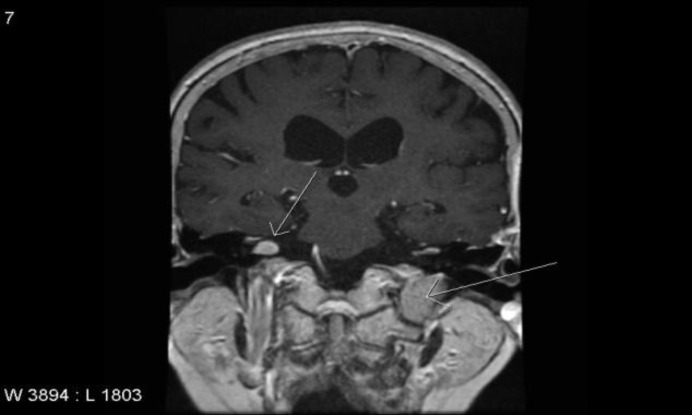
Coronal magnetic resonance imaging demonstrating a right-sided sporadic vestibular schwannoma (short arrow) and a left-sided glomus jugulare tumor (long arrow)
